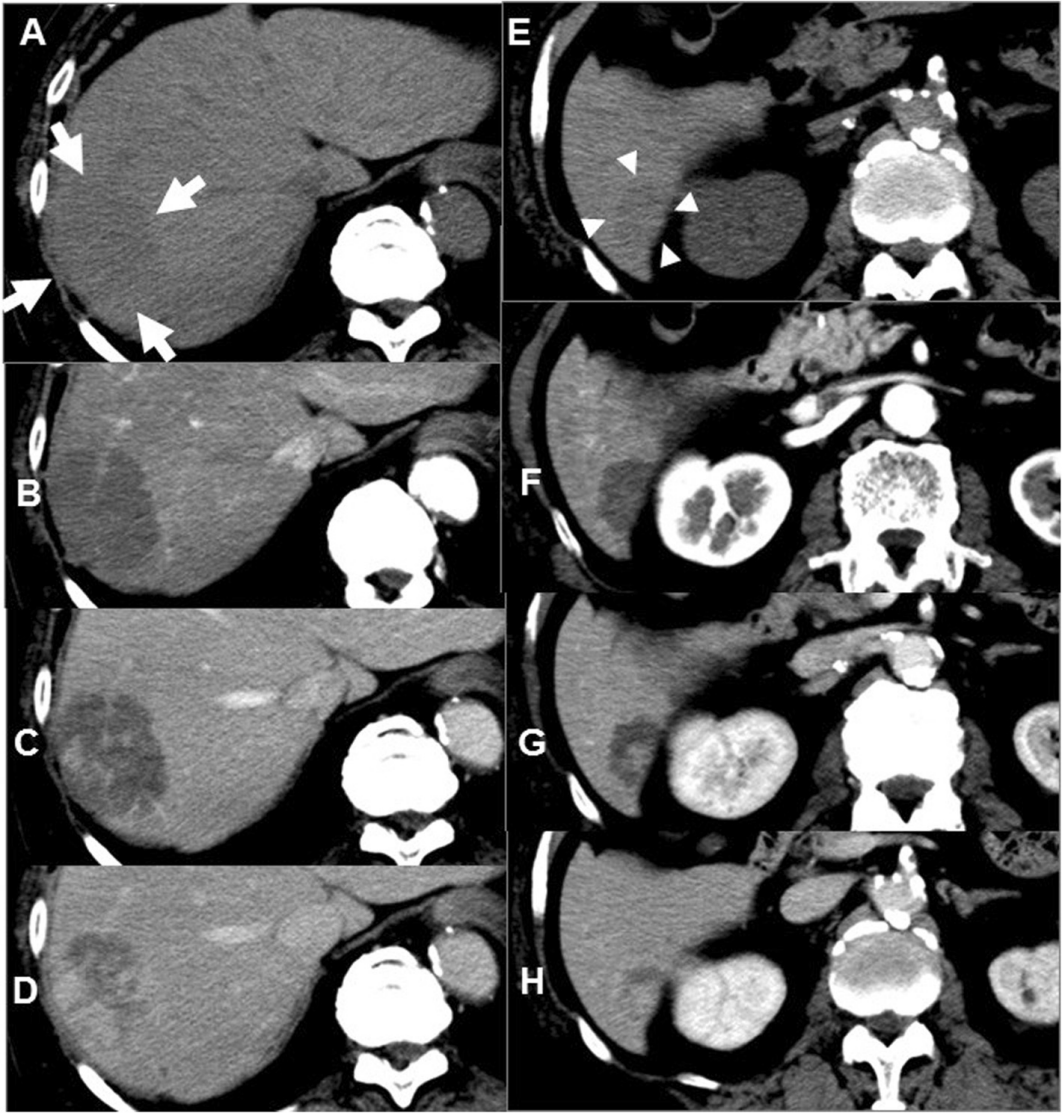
Abdominal computed tomography imaging (a–d: segment 7, e–h: segment 6). a (white arrows), e (white arrowheads) Plain phases. b, f Arterial phases. c, g Portal phases. d, h Delayed phases. a, e Both tumors are isodense before contrast injections, (b, f) and show hypoenhancement in the arterial phase. c, g In the portal venous phase, the tumor in segment 7 shows heterogeneous enhancement, and the tumor in segment 6 shows central enhancement. d, h In the delayed phase, the tumor in segment 7 shows more heterogeneous enhancement, and the tumor in segment 6 shows more central enhancement
